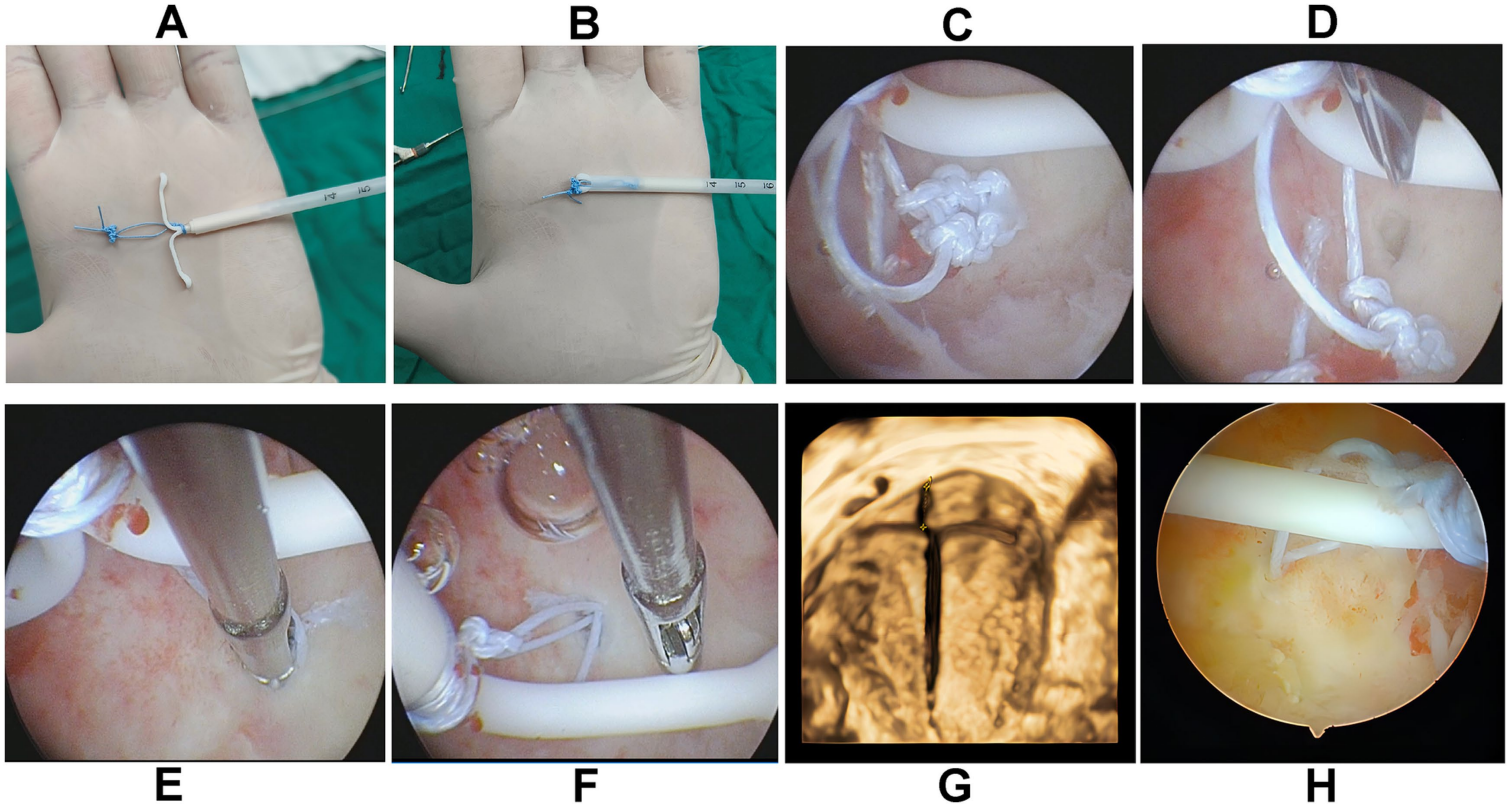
Hysteroscopic sutureless fixation of the LNG-IUS. (A,B) A non-absorbable wire knot is made at the upper end of the LNG-IUS, which is then retracted into the casing. (C) The LNG-IUS was inserted into the uterine cavity. (D–F) A small incision was made in the myometrium using scissors, and the knot head of the LNG-IUS was inserted into the myometrium using hysteroscopic forceps. (G) A three-dimensional ultrasound confirmed that the wire knot head was securely fixed within the myometrium of the uterine fundus. (H) A follow-up hysteroscopy examination after 1 month showed that the small incision in the myometrium had healed and the LNG-IUS had been properly fixed.
